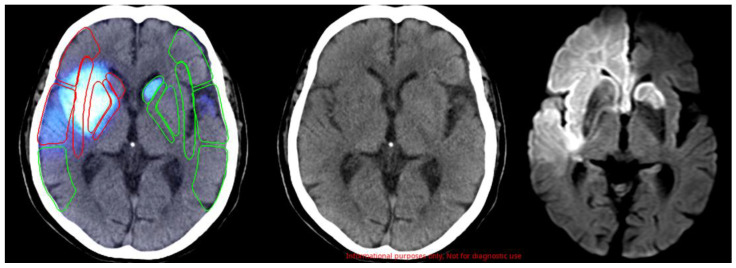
Representative images of ischemic stroke by AI software. From left to right: images assessed using the AI software, non-contrast head CT scan, and diffusion-weighted head MRI. In the AI software image, the red framed areas indicate early ischemic changes and the green framed areas indicate non-ischemic changes.
